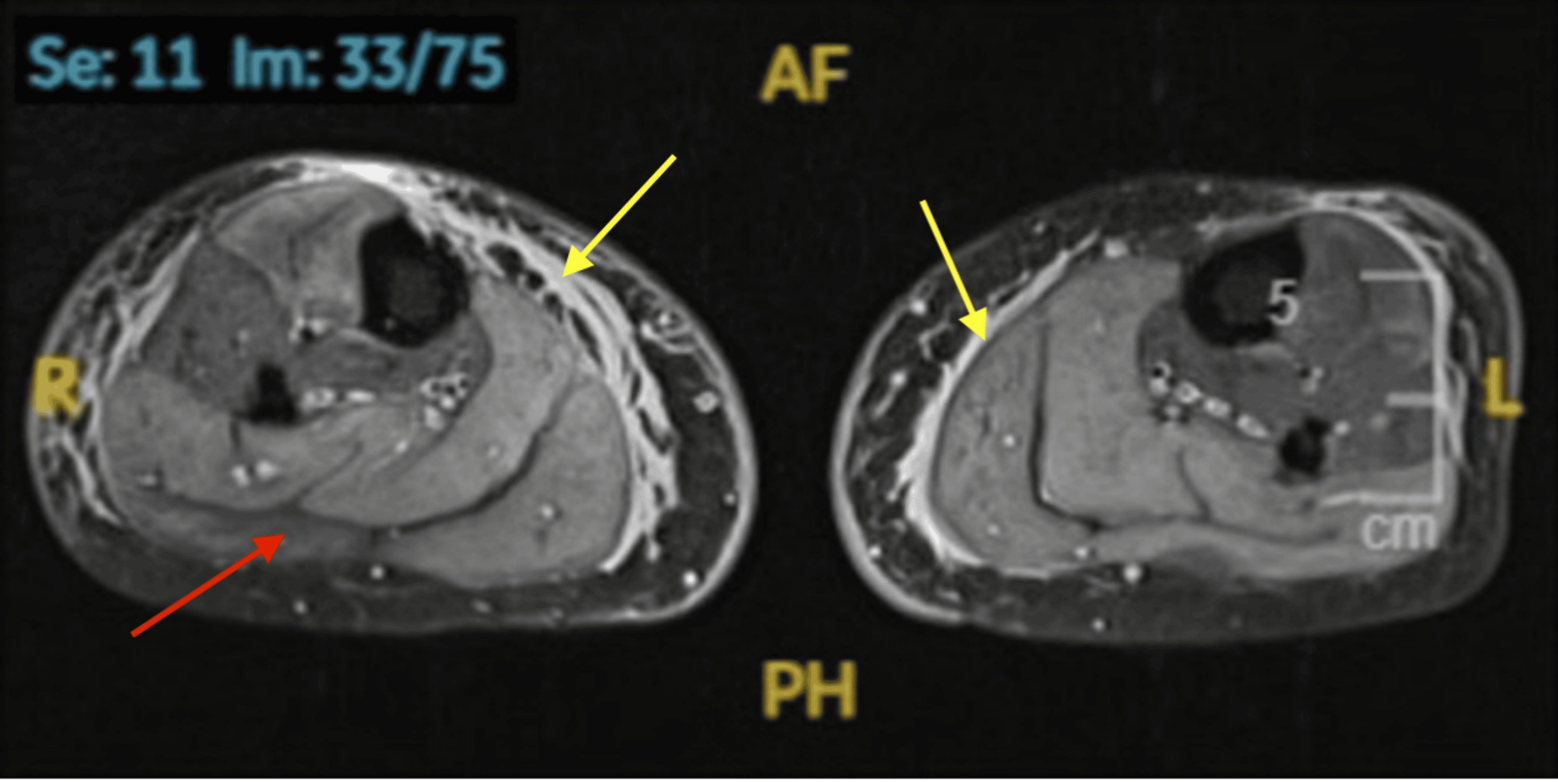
MRI cross-sectional STIR sequence images of the right and left thighs demonstrating generalized bilateral symmetrical muscle edema, suggestive of PM The yellow arrow indicates subcutaneous tissue edema, and the red arrow indicates intermuscular edema. PM, polymyositis; STIR, short tau inversion recovery
